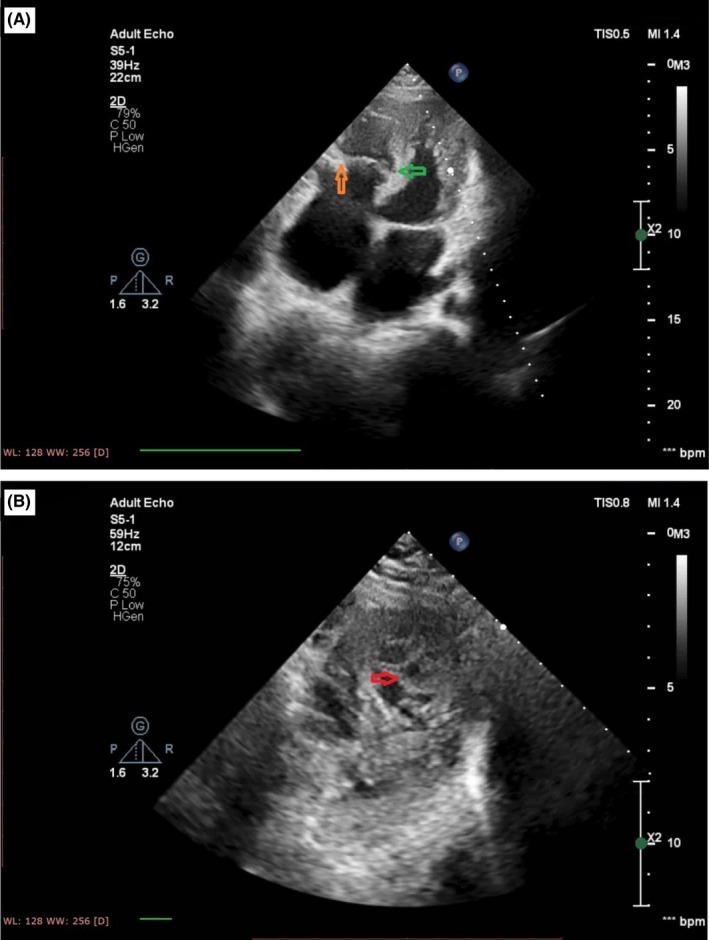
Transthoracic echocardiography. (A) Four‐chamber view showed apical displacement of all three tricuspid valve leaflets with no anterior leaflet elongation. (B) Parasternal short axis view showed apical non‐compaction of left ventricle as an associated anomaly
